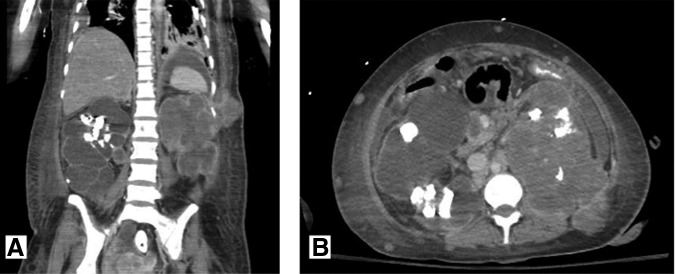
Case 2: CT abdomen and pelvis from December 2015 (A: coronal, B: axial) demonstrating marked progression of metastatic disease, including metastatic lesions of the lung, pleura, pancreatic neck, and innumerable lesions in the subcutaneous tissue.
